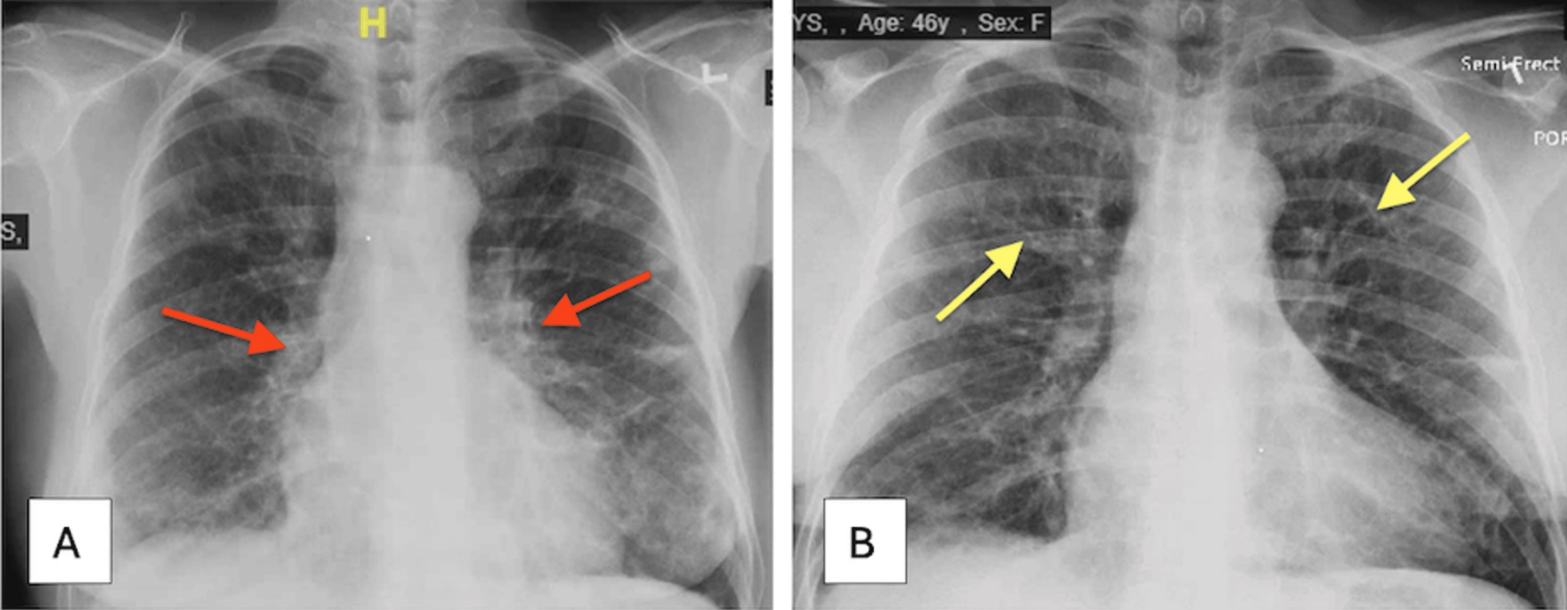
Chest X-ray showing (A) generalized diffuse vascular markings (red arrows) and (B) chronic interstitial lung changes (yellow arrows).
